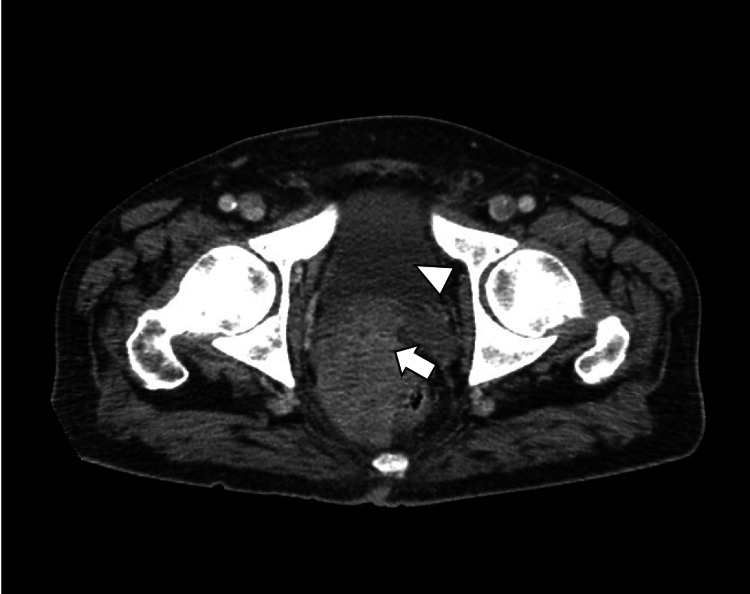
CT scan (transverse view) of the abdomen and pelvis White arrowhead shows urinary bladder, and white arrow shows tumor arising from the prostate compressing the base of the urinary bladder and extending posteriorly towards the rectum CT, computed tomography
